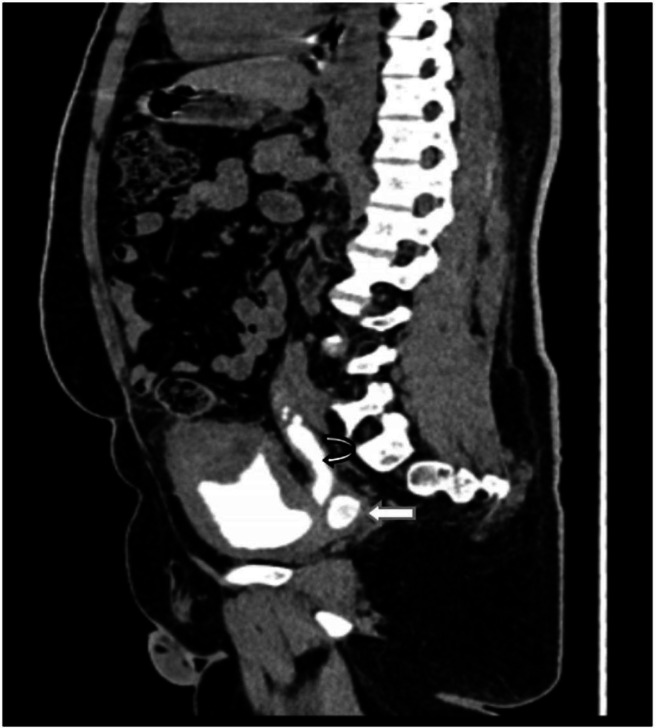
Image of excretory phase of CT urogram in sagittal plane showing left primitive ureter draining into left bladder compartment (annotated by curved white arrow) with a VUJ diverticulum at the draining point (depicted by white arrow).
